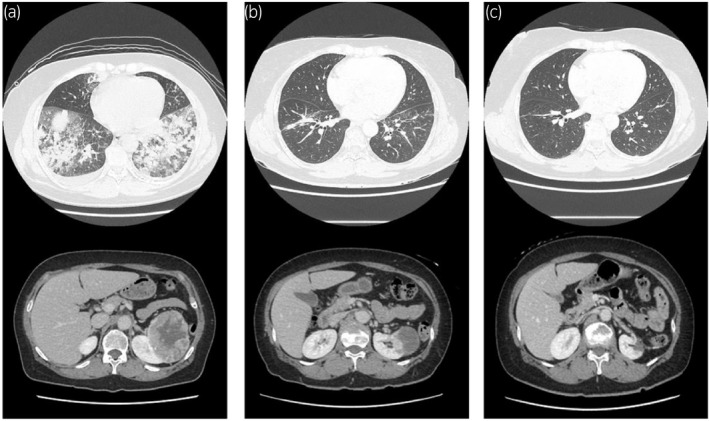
CT images. (a) At the time of initial presentation, bilateral infiltrative shadows can be seen in both lungs and a 85 × 83 mm mass with contrast enhancement at the upper pole of the left kidney. (b) After ICI treatment before surgery, showing CR for the metastasis in the lung and PR for the renal tumor. (c) One year after CPN. There is no local or distal recurrence.
